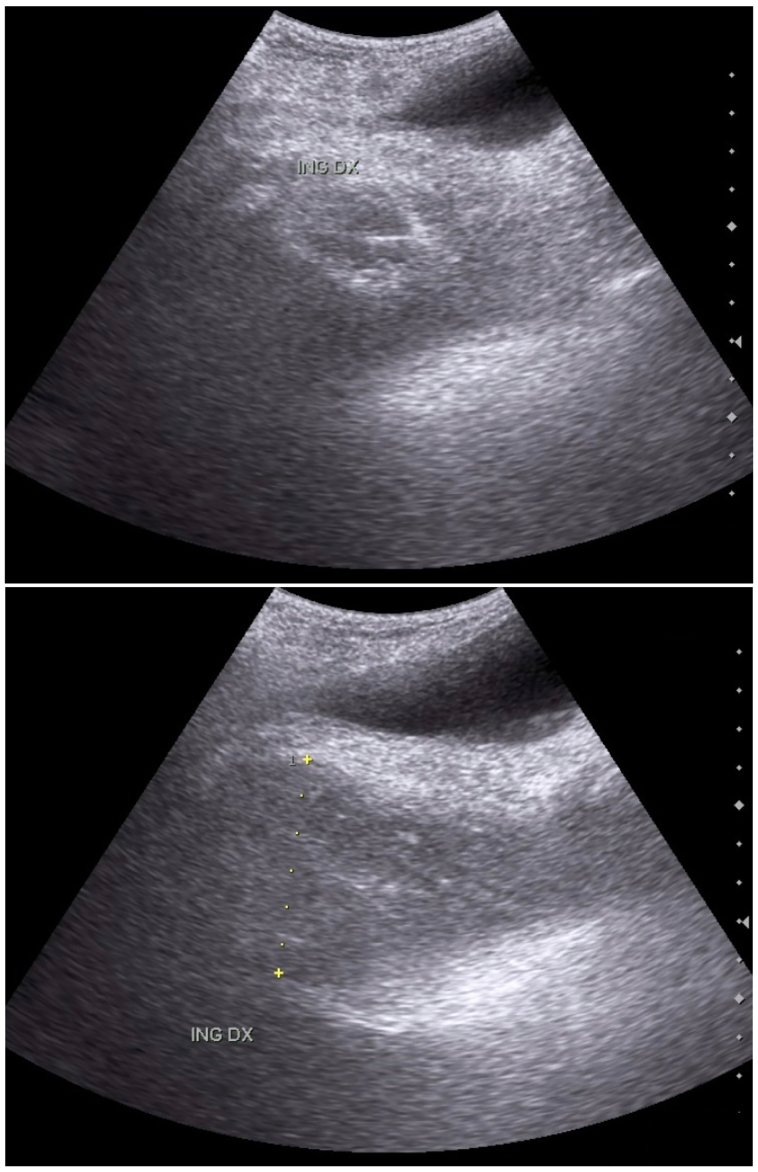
Abdominal and pelvis ultrasonography (US) revealed widened inguinal canal containing peritoneum and “teardrop” lesion at the inguinal continuing until the right scrotal.
